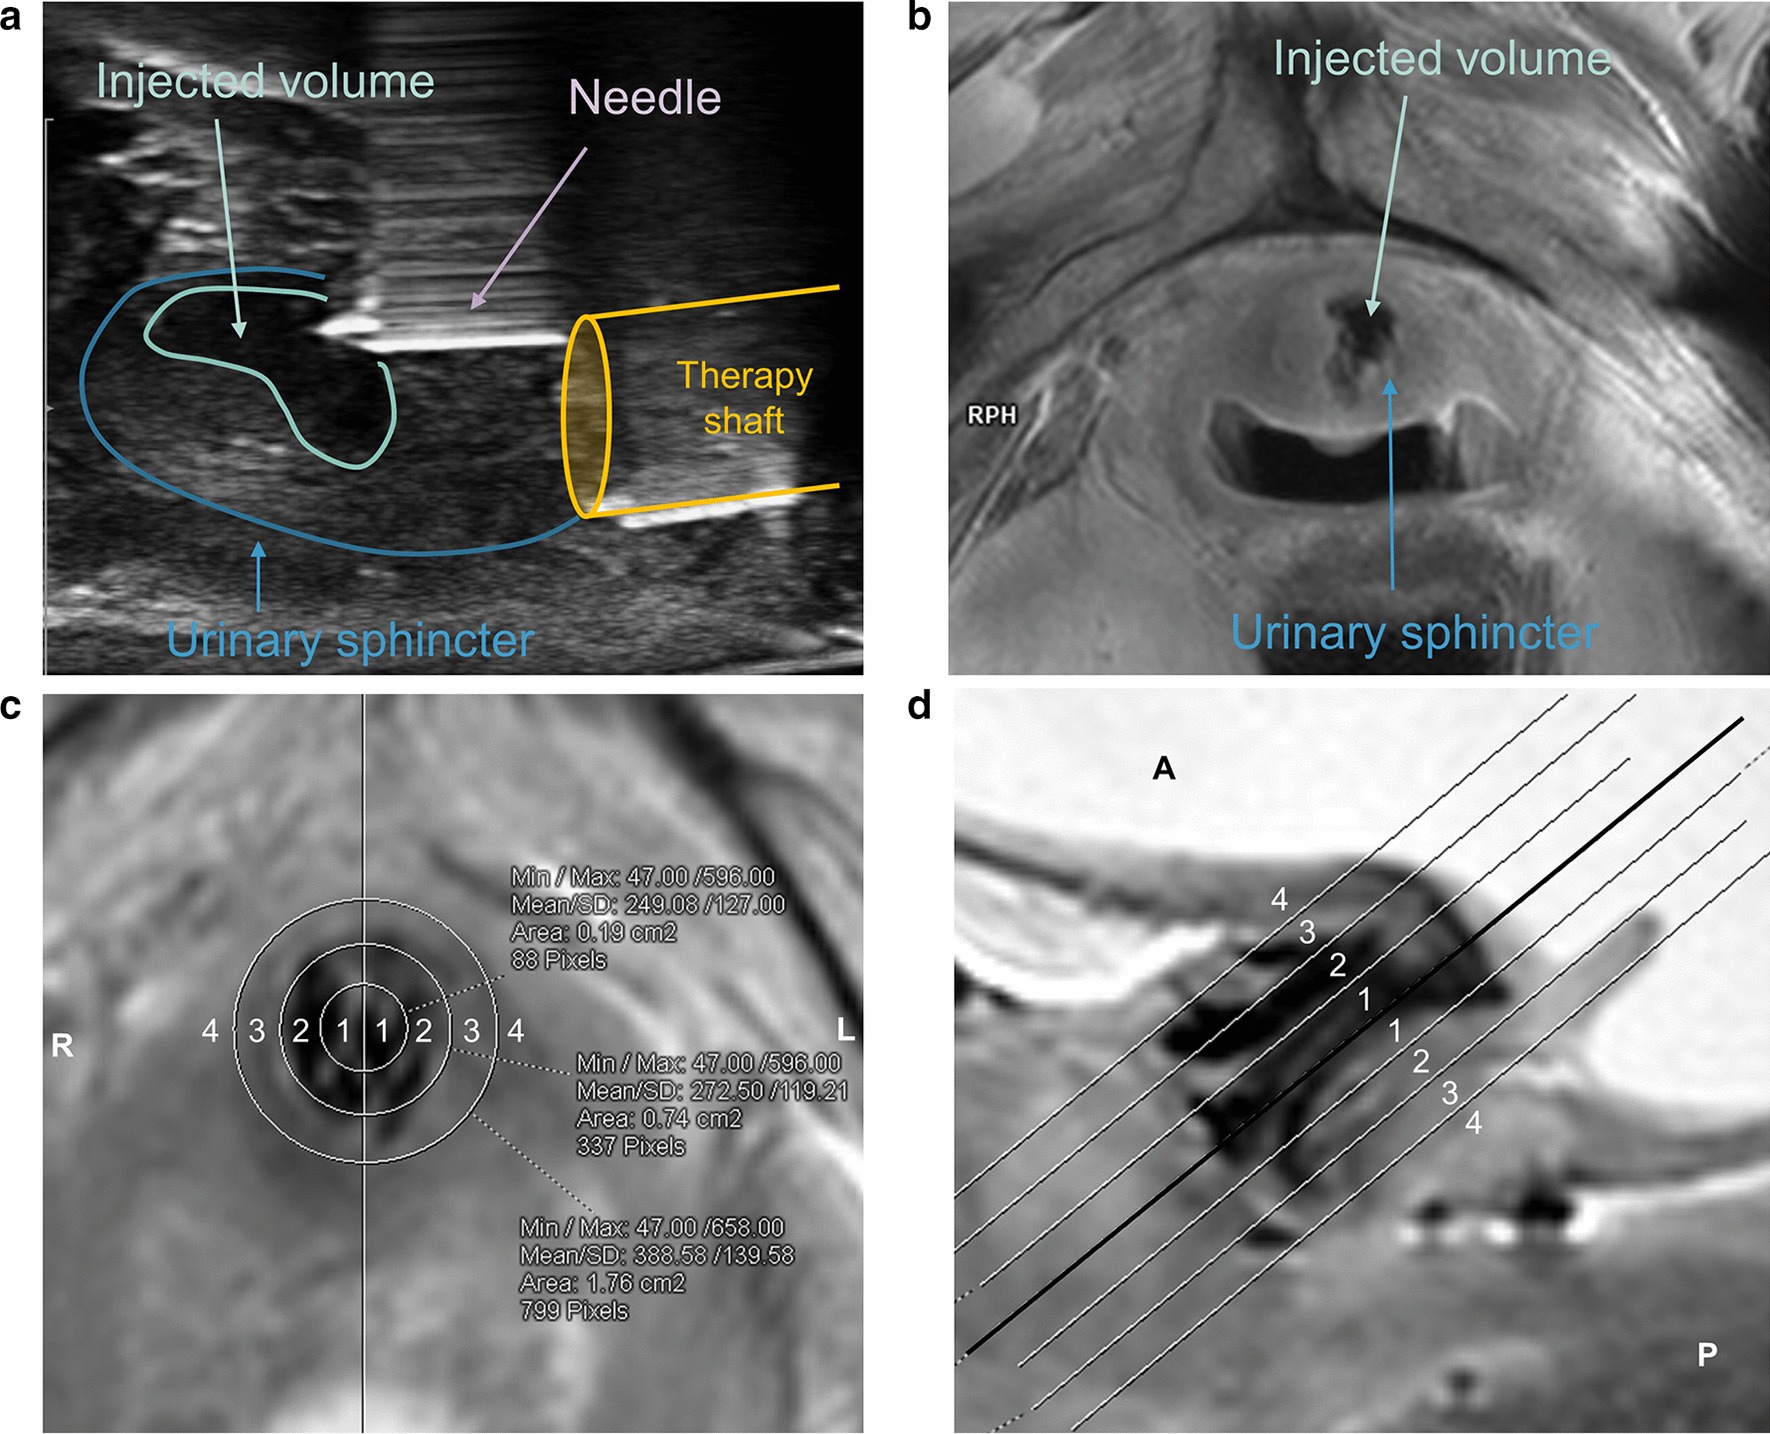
a US image in sagittal orientation during transurethral injection. b MRI image in axial orientation after injection of nano-iron particles. c, d Scoring of injection defined on a rating scale from 1 to 4 points according to extinction signal of injected iron nano-particles in MRI images. c Axial orientation with division into right and left, d sagittal orientation with division into anterior and posterior. Diameter and distances in (a) and (b), respectively: 1 = 5 mm, 2 = 10 mm, 3 = 15 mm, 4 > 15 mm
